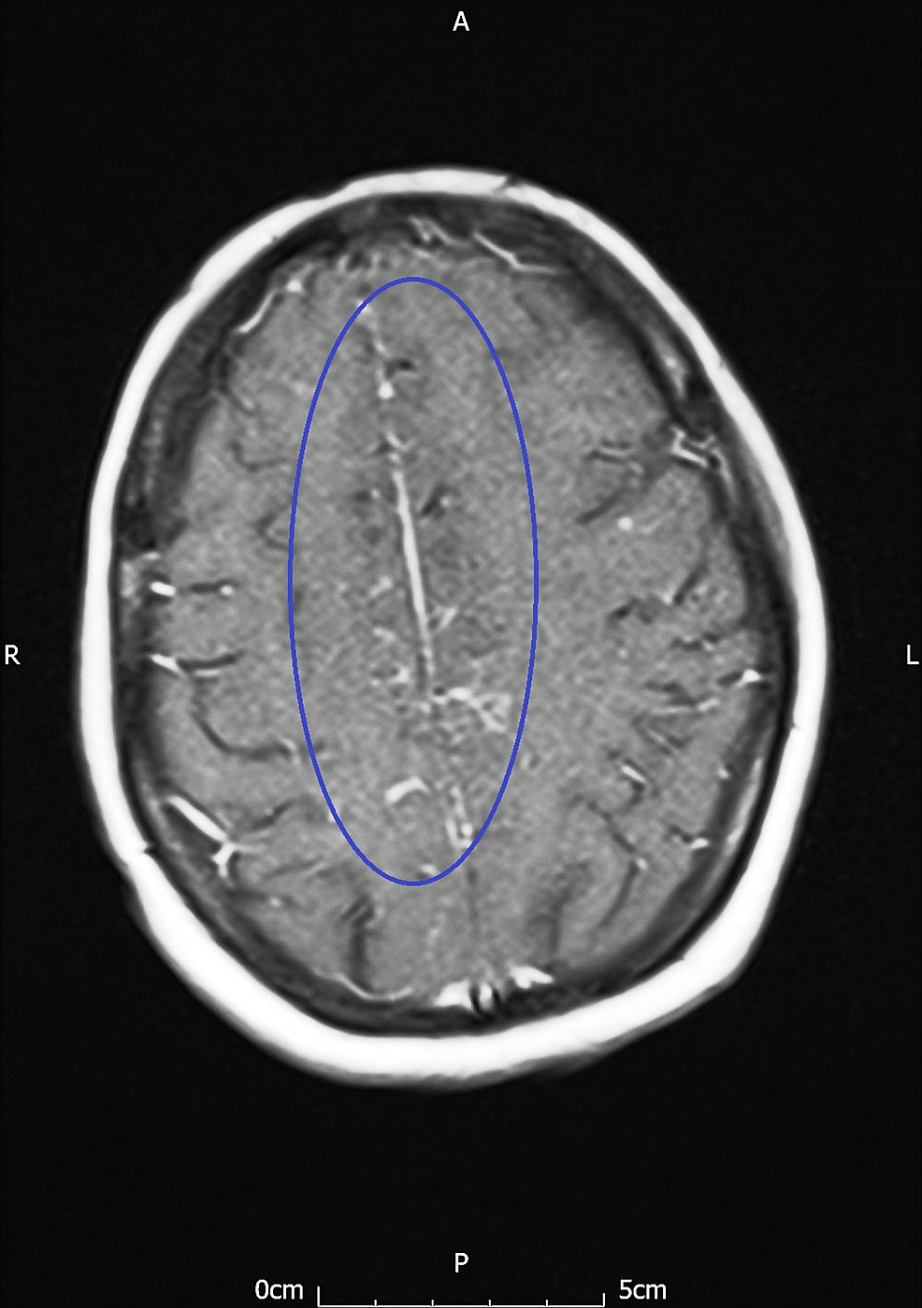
T1W axial post-contrast MRI head showing leptomeningeal enhancement in the parafalcine cerebral hemisphere.
